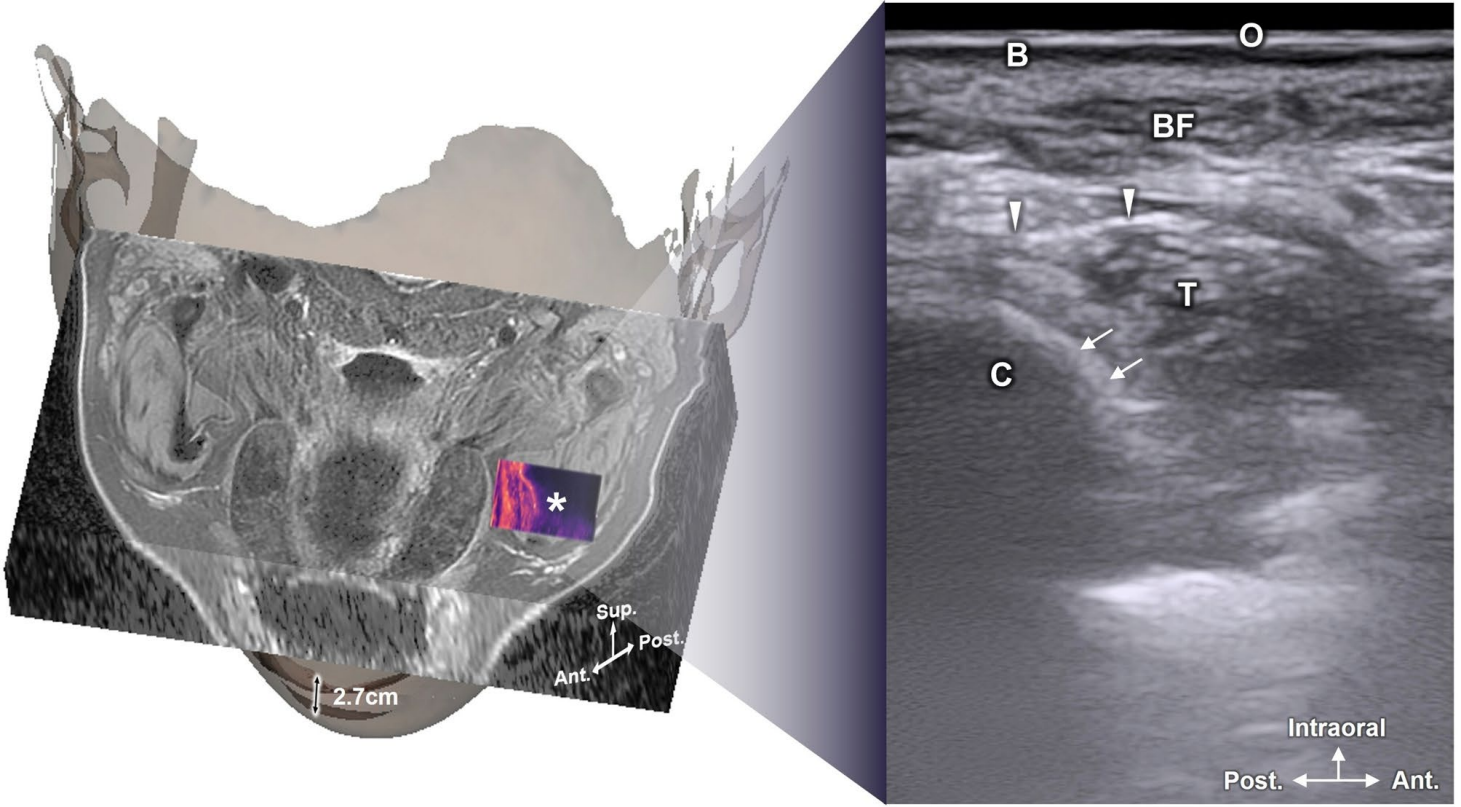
Superimposed model of the magnetic resonance imaging and a three-dimensional scanned face with tracked intraoral ultrasonography image. Ant, anterior; Post, posterior; Sup, superior; Asterisk, tracked US image; C, coronoid process; T, temporalis muscle; B, buccinator muscle; BF, buccal fat pad; O, oral mucosa; arrow, anterior border of coronoid process; arrowhead, temporalis tendon.
